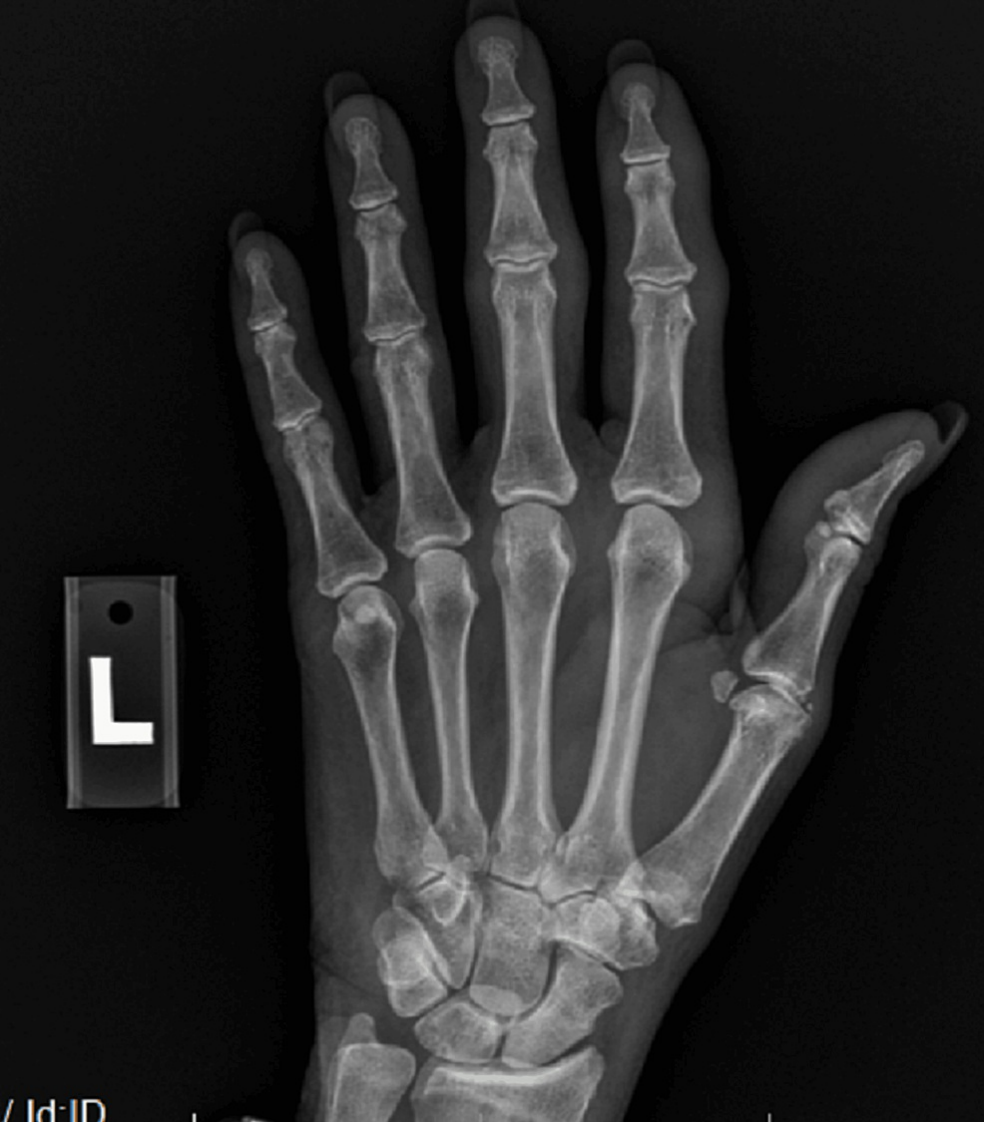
Radiographic examination of the hands indicates a reduction in joint space observed at the first CMC; PIPs, and DIPs​ CMC: carpometacarpal, PIP: proximal interphalangeal joint, DIP: distal interphalangeal joint
